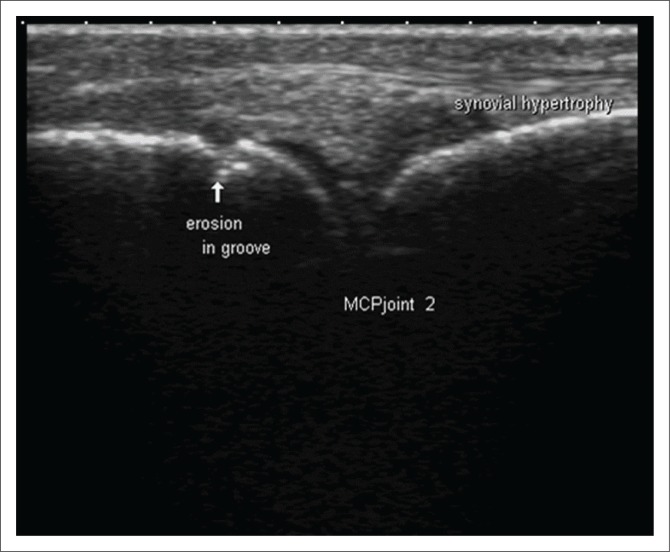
Longitudinal ultrasound at the level of the second metacarpophalangeal joint shows synovial hypertrophy with an early erosion.
